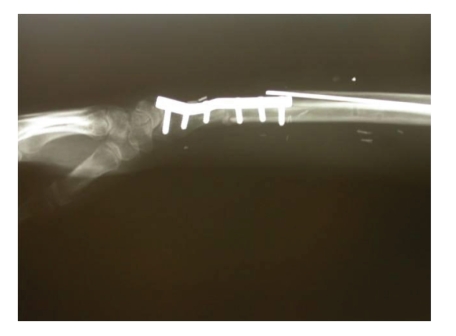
X-ray showing a reduced fracture with plates and screws.
